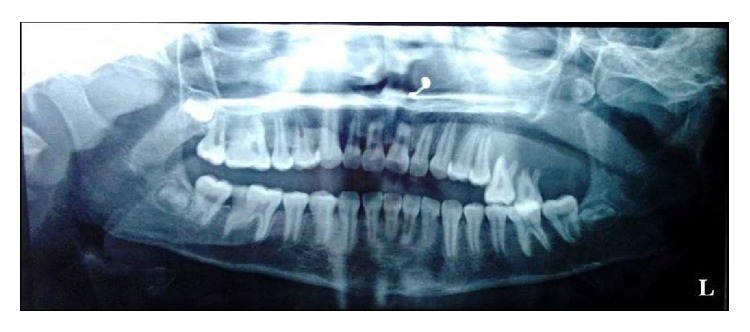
Orthopantomogram showing displaced molars with resorption of roots.
